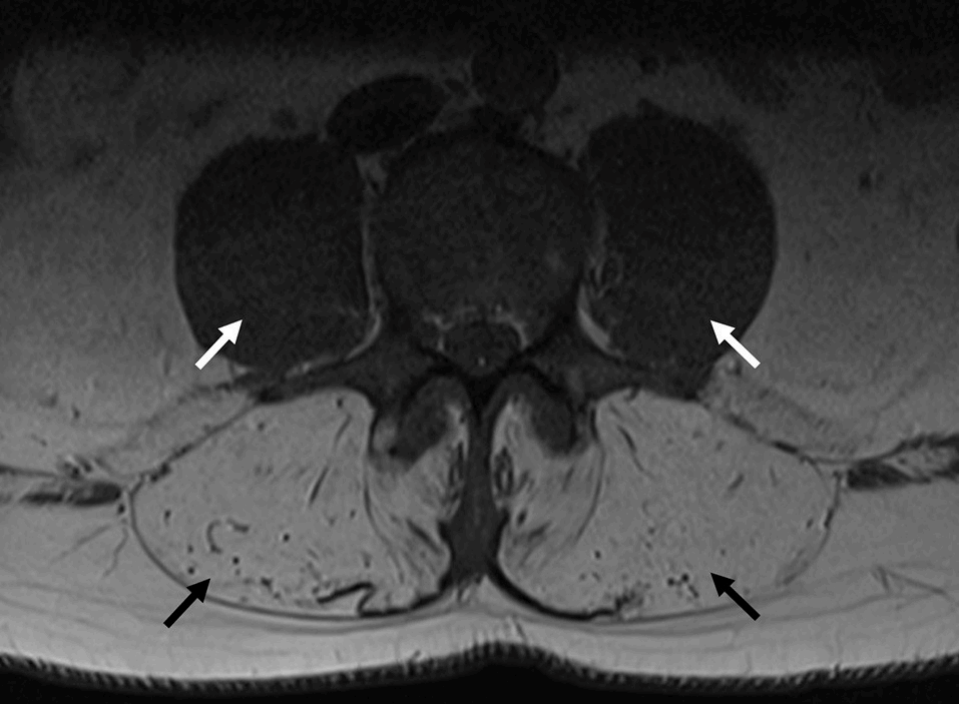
MRI of the lumbar spine from Proband 2. Transverse axial image of a T1 sequence in the lumbar spine, indicating severe paraspinal muscle atrophy (black arrows), with preservation of psoas muscles (white arrows).
